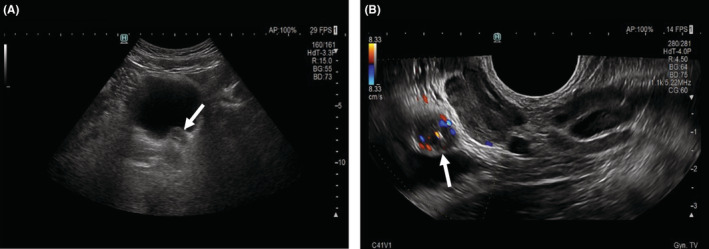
Ultrasonography of small cell neuroendocrine carcinoma of the ureter. (A) Transabdominal ultrasonography showed a hypoechoic mass located in the lower ureter (arrow) that was uniform on internal echo with an undefined boundary. (B) Transrectal ultrasonography showed that the blood flow of the mass was abundant (arrow), and the ureter wall around it was abnormal.
